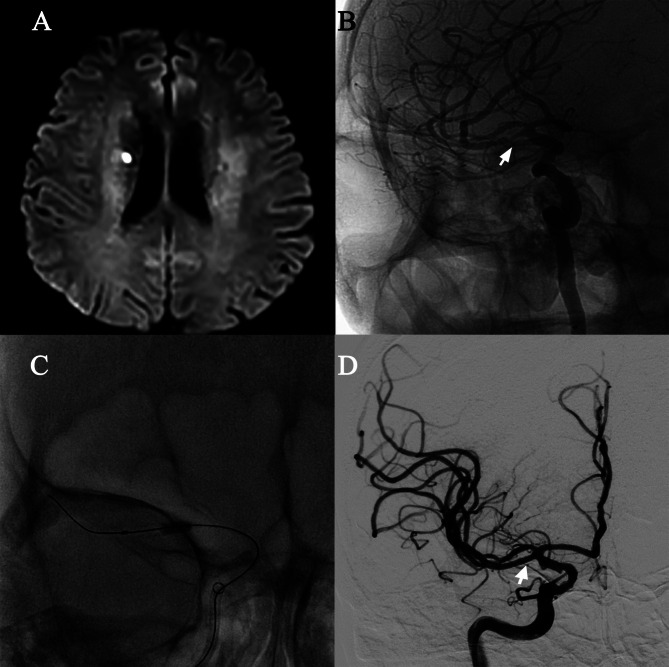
(A) DWI-sequence axial MRI showed a new infarct in the right corona radiata. (B) 3D-DSA imaging. (C) The stent was successfully released at six atmospheric pressures. (D) The stenosis disappeared after the DMCA stent implantation (a 2.25/10 mm NOVA intracranial sirolimus-eluting stent system, SINOMED, Tianjin, China)
